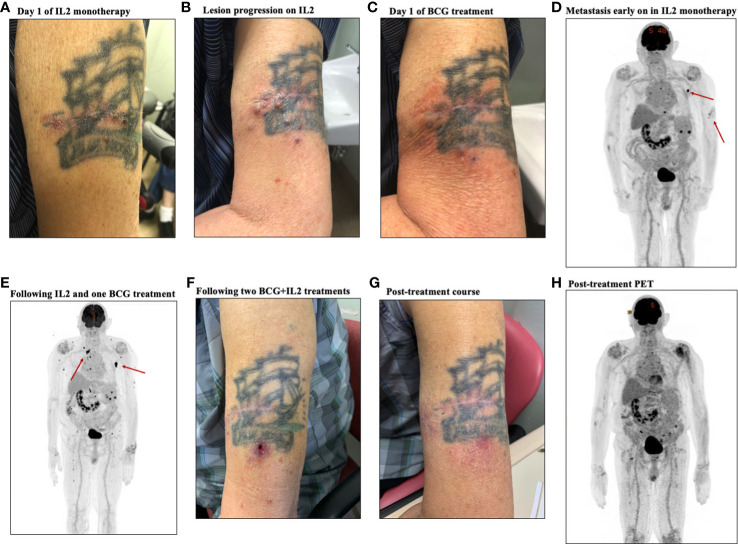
Representative clinical course of a case of a complete responder, patient #2. (A) Photo of primary lesion on day one of IL-2 treatment. (B) Photo of lesion following IL-2 monotherapy showing progression of lesions. (C) Photo of lesion on the day of BCG initiation, showing stable disease (D) PET-CT early on during IL-2 monotherapy showing extent of disease, with metastatic node highlighted. (E) PET-CT following treatment with IL-2 and one BCG treatment, showing metastasis. (F) Photo of the lesion following two BCG treatments, showing the typical ulceration seen with BCG injection. G. Photo of the treated area following treatment course, showing complete resolution. H. Post-treatment PET CT, showing reactive lymphadenopathy with no further evidence of metastatic disease and radiographic resolution of the cutaneous lesions.
